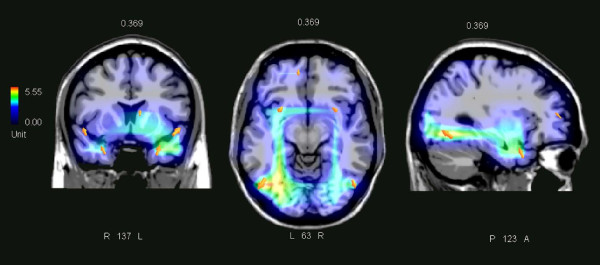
swLORETA [19] inverse solution displaying the neural generators of the N3 suppression effect for pseudo-targets. LORETA was computed on the difference wave obtained by subtracting ERPs to L-F+ from ERP to pseudo-targets in the time window 370–430 ms, corresponding to the maximum amplitude of the prefrontal N3 response. A realistic boundary element model (BEM) was derived from a T1 weighted 3D MRI data set by segmentation of the brain tissue. The BEM model consisted of one homogenic compartment made up of 3446 vertices and 6888 triangles. The head model was used for intra-cranial localization of surface potentials. Segmentation and head model generation were performed using the ASA (A.N.T. Software B.V., Enschede, the Netherlands) package [45]. The electromagnetic dipoles are shown as arrows and indicate the position, orientation and magnitude of dipole modelling solution applied to the ERP difference wave in the specific time window (370–430 ms). The different colours represent differences in the magnitude of the electromagnetic signal (in nA). 0.369 indicates the boundary of time window (370 ms at 512 Hz of sampling rate). L = left; R = right; P = posterior; A = anterior; numbers refer to the displayed brain slice in coronal, axial and sagittal views, respectively.
